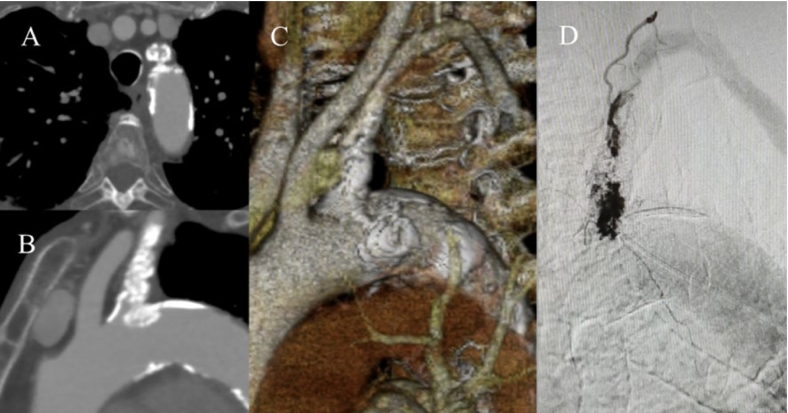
Pre-operative computed tomography findings. The axial (A), sagittal (B), and three-dimensional images (C) reveal a severely stenotic lesion with calcification of the left subclavian artery. The pre-operative angiography image reveals that the axillary artery drains via the collateral circulation (D).
